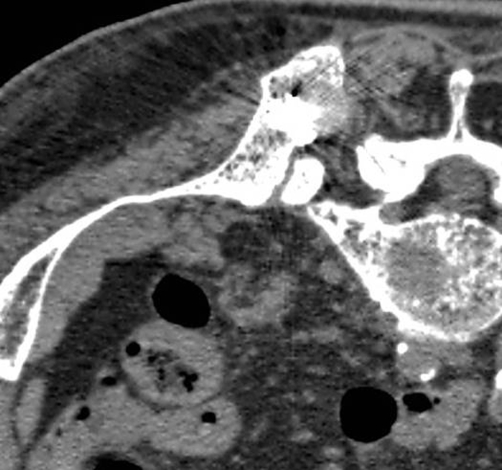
CT scans after the treatment. CT scans shows the distribution of cement inside the cavity created into the lesion.
